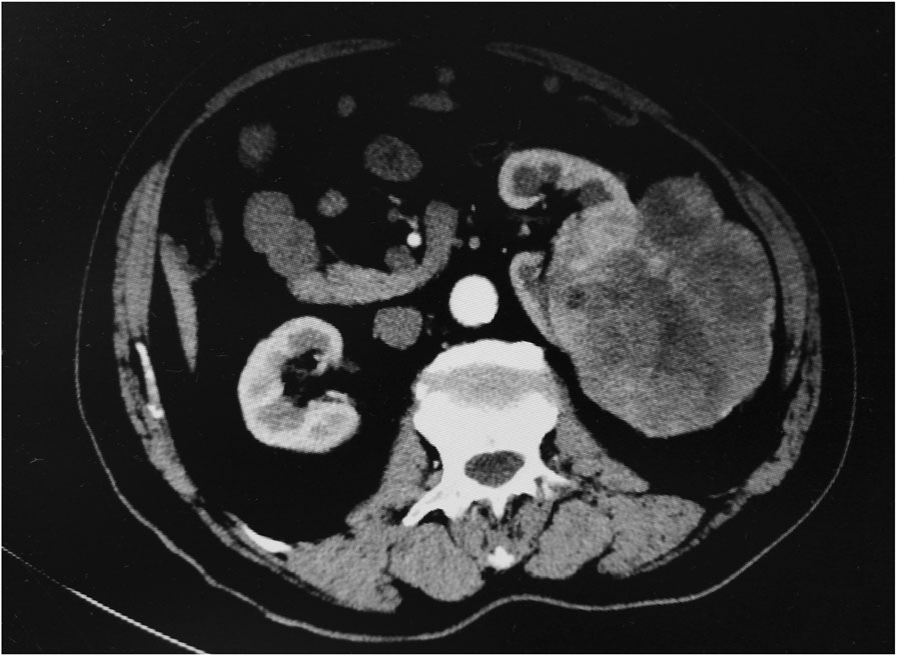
Abdominal CT showing a tumour in the left kidney
